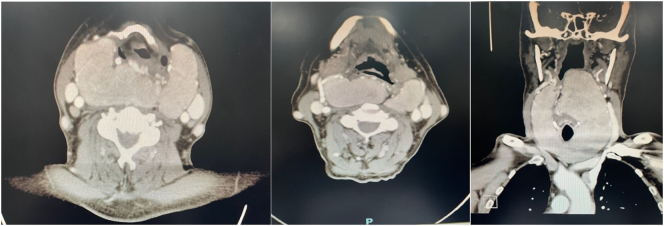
Large retropharyngeal goiter seen in imaging. CT scan of the neck revealing a large thyroid goiter. Right hyoid bone fracture is noted.
